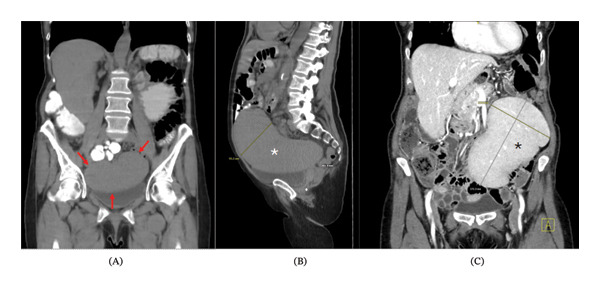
Abdominal computed tomography (CT) suggestive of a pelvic tumor measuring 20 × 9.5 × 13 cm (Panel (A) with red arrows, Panel (B) with white asterix, Panel (C) with the black asterix). Panels (A) and (B) are from the CT abdomen/pelvis performed in April 2024, and Panel (C) is from the CT abdomen/pelvis performed in June 2024.
